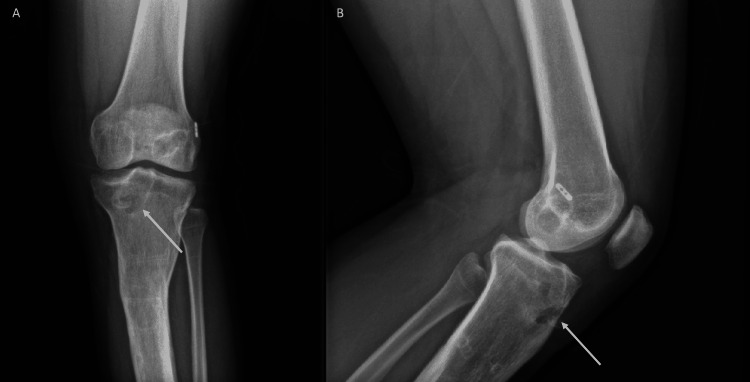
Anteroposterior and lateral X-rays of the pretibial cystic lesion prior to curettage and grafting. A) Left knee anteroposterior view showing the pretibial cyst; B) left knee lateral view showing the pretibial cyst.
